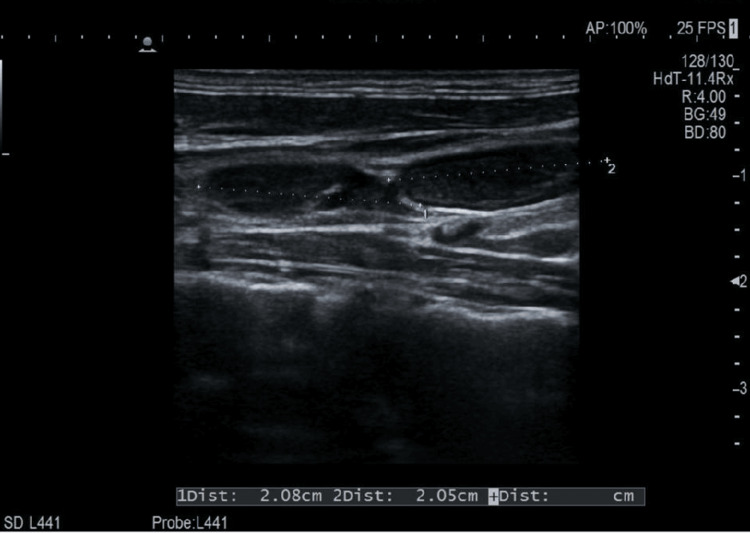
Longitudinal cervical lymph node ultrasound Longitudinal ultrasound image of a left-sided cervical lymph node (level IV) measuring approximately 2.08 × 2.05 cm.
